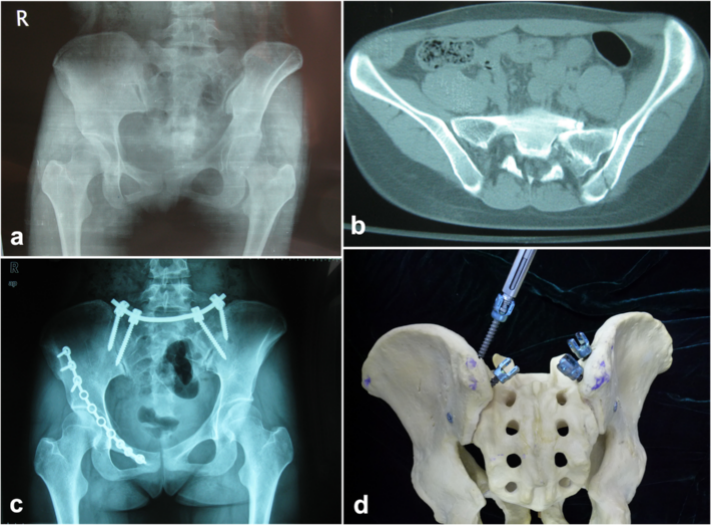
Patient with closed unstable pelvic ring disruption. A 43-year-old woman had a type B (Tile classification) pelvic fracture caused by a traffic accident. a Preoperative pelvic radiographic image. b Preoperative computed tomographic image. c Postoperative pelvic radiographic image. d Pelvic model demonstrates two pedicle screws in each dorsal iliac crest
